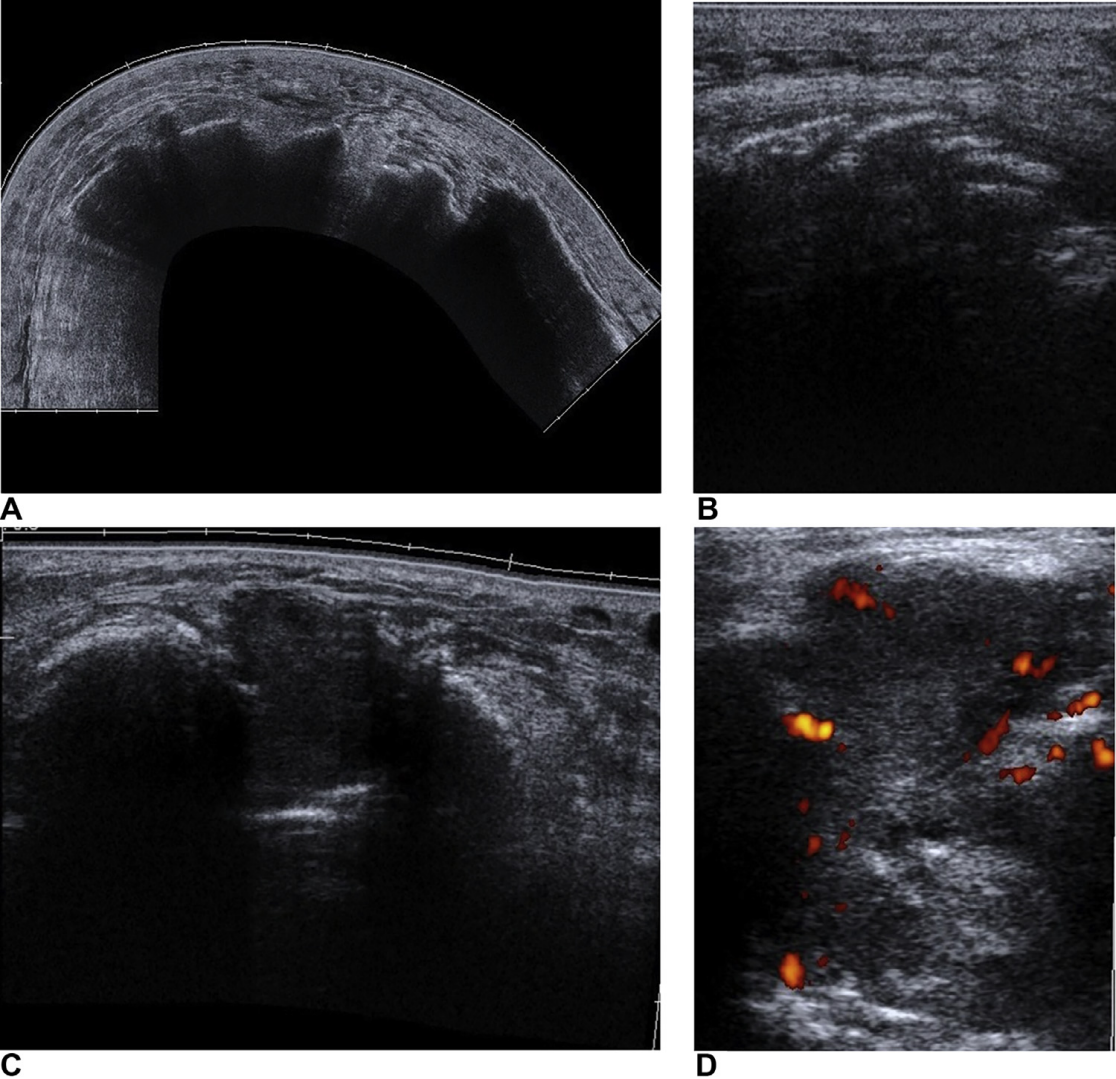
Follow-up ultrasonography showing well-demarcated, calcified mass with acoustic shadowing in the central part of left calf (A), spreading to the adjacent muscle along the muscle fascicle (B), almost anechoic soft-tissue area in the superficial part of the mass (C), distinctly vascularized (D), accessible to biopsy.
